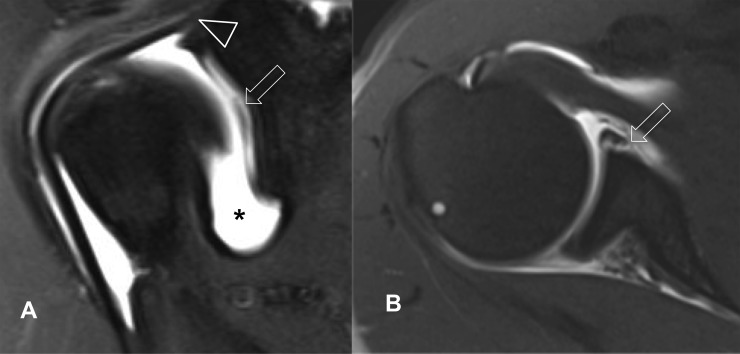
Coronal fat saturated proton density (A) and axial fat saturated T1-weighted (B) MRA of the right shoulder in a 45-year-old male tennis player. There is an extensive superior labral anterior to posterior (SLAP) tear (open arrow), while the biceps anchor (arrowhead) is still intact. Intraarticular contrast agent (*) has been applied prior to the examination.
